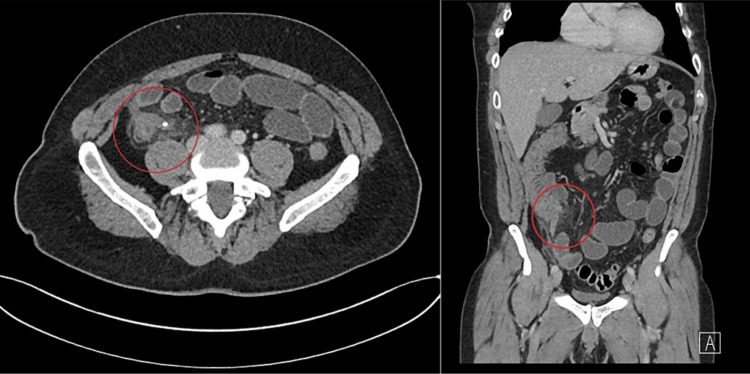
CT of the abdomen and pelvis with contrast confirmed acute appendicitis
